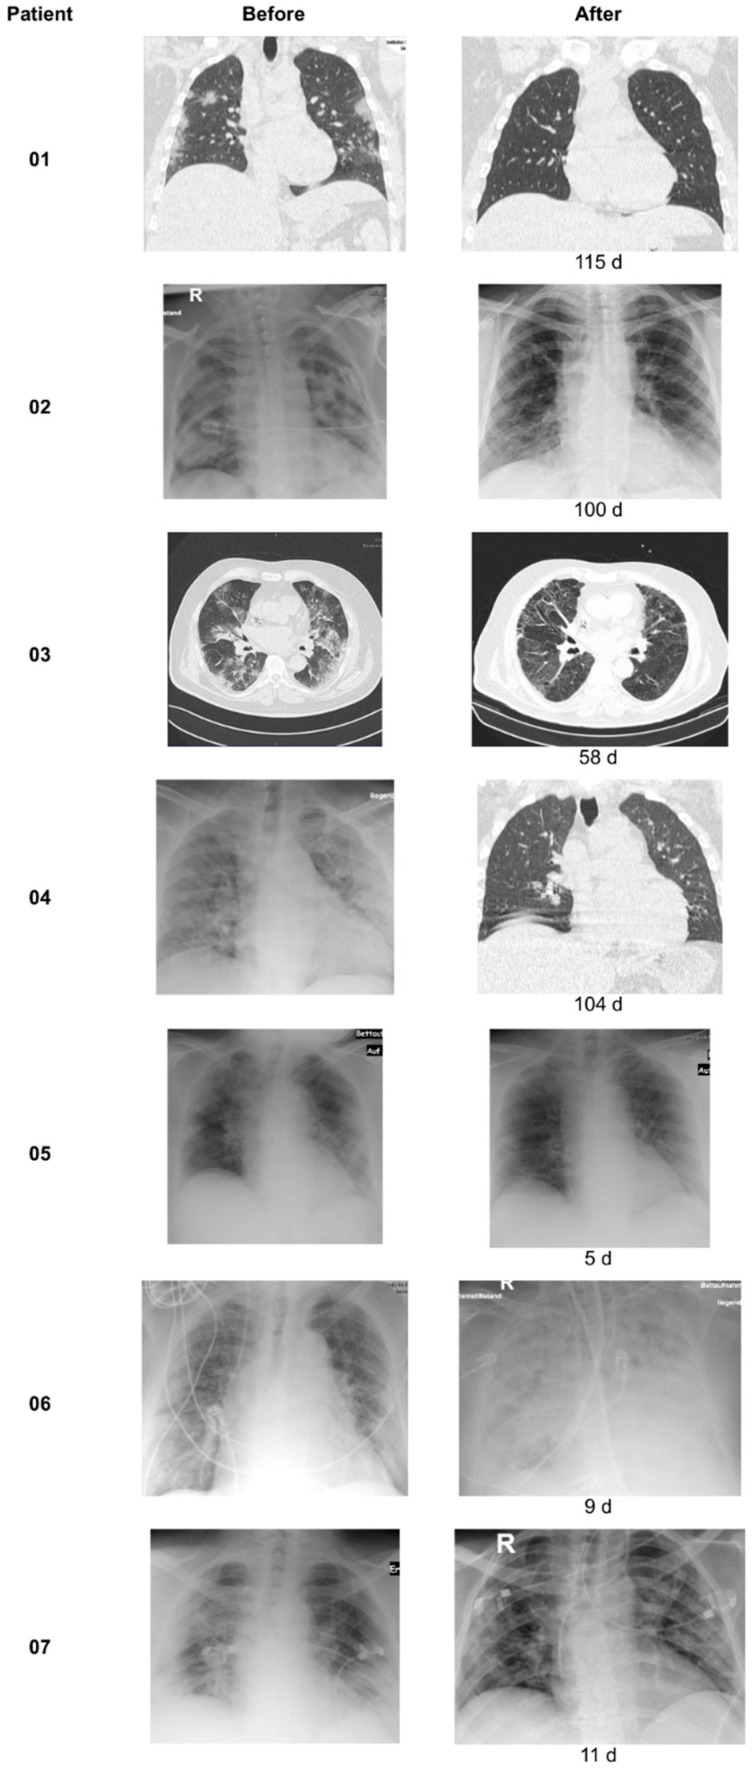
X-ray/CT chest scans. Chest scans were performed before and after treatment/as follow up. The time (days) between the different scans is indicated at the second chest scan.
